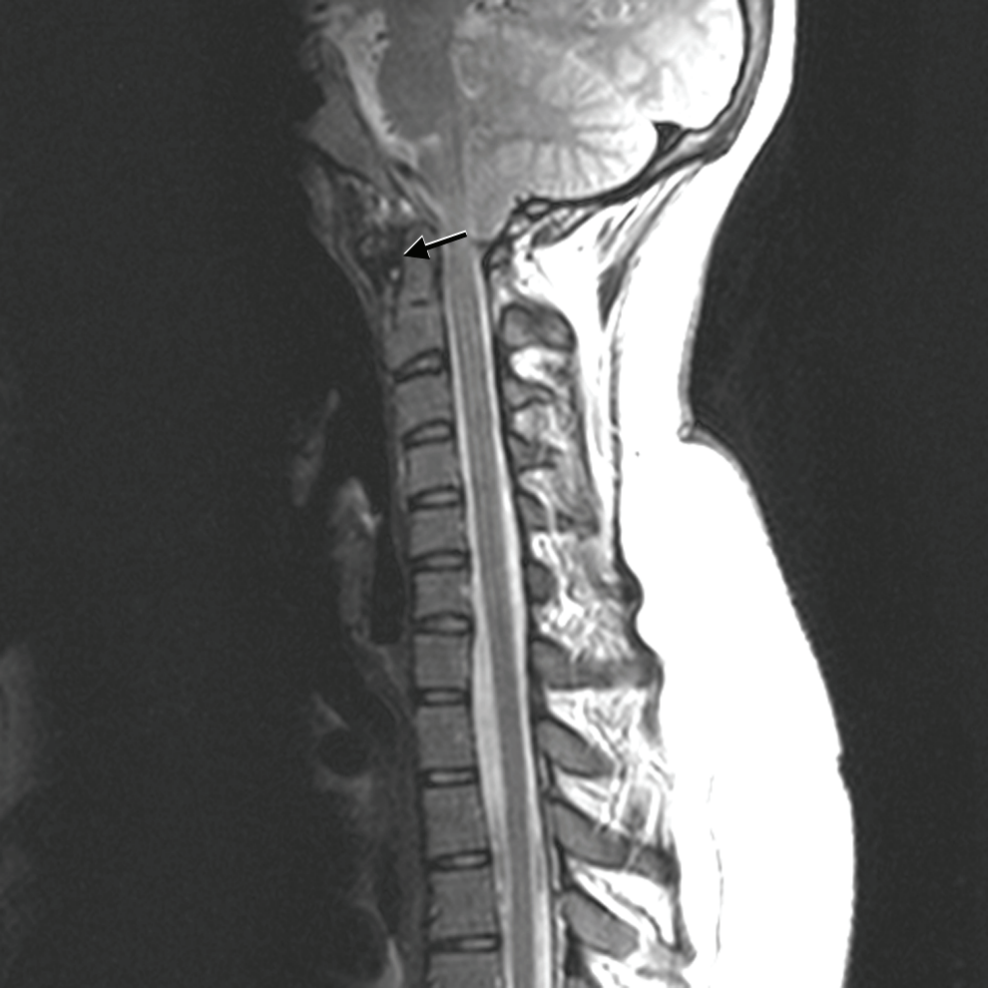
Sagittal T2-weighted MRI of the cervical spine demonstrates widening of the atlantodental interval, cervical canal stenosis without spinal cord signal changes, and pannus formation (arrow).
